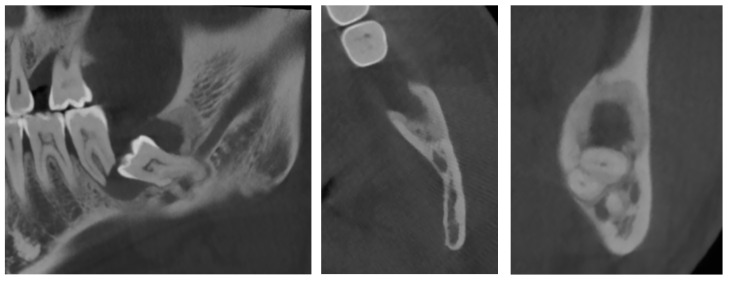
CBCT X-ray of the case #3 patient.
